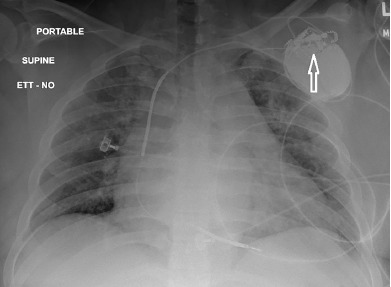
Anterior posterior chest radiograph showing cardiomegaly and damaged header of the automated implantable cardioverter defibrillator (arrow).
